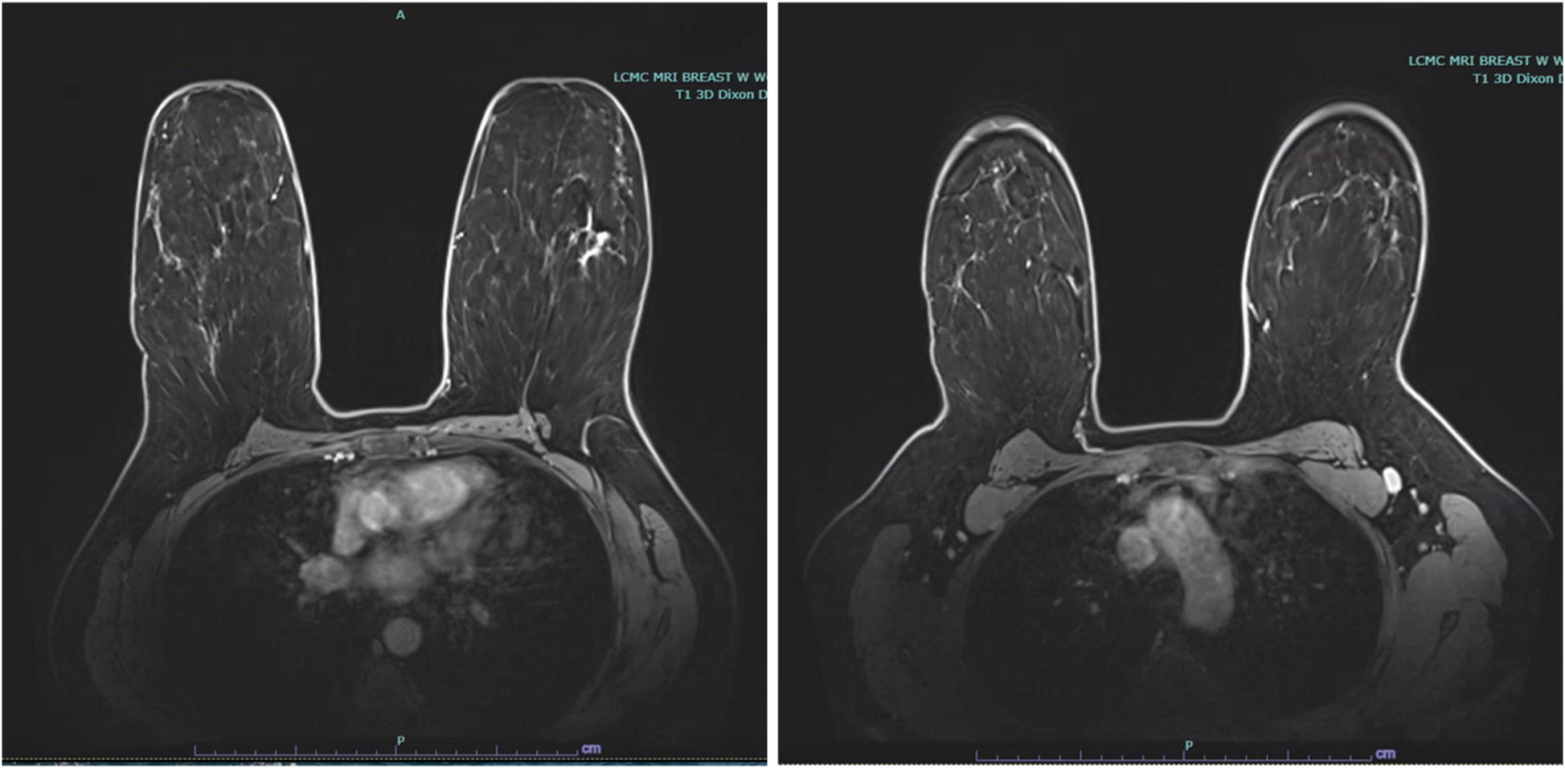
Bilateral breast MRI depicting malignancy in the upper outer quadrant of the left breast with non-mass enhancement measuring 5.2 cm, in addition to a prominent level 1 left axillary lymph node.
